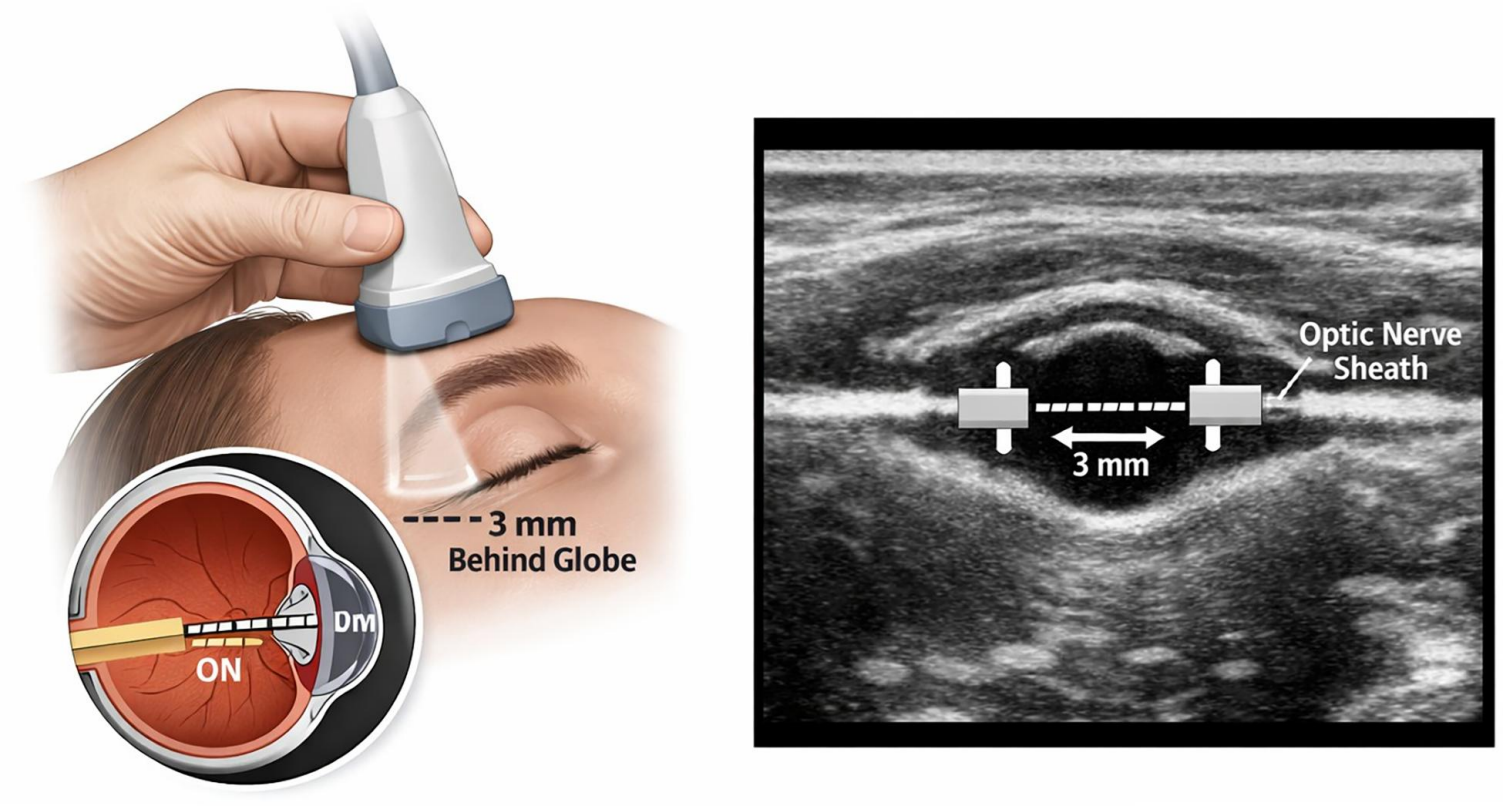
Ocular ultrasonography (OUS) and measurement of the optic nerve sheath diameter. OUS offers a non-invasive, real-time, portable, and low-cost alternative to other methods, such as CT or MRI which lacks real-time capability, or invasive ICP monitoring which is associated with procedural risks and limited practicality during epiduroscopy despite providing continuous data.
